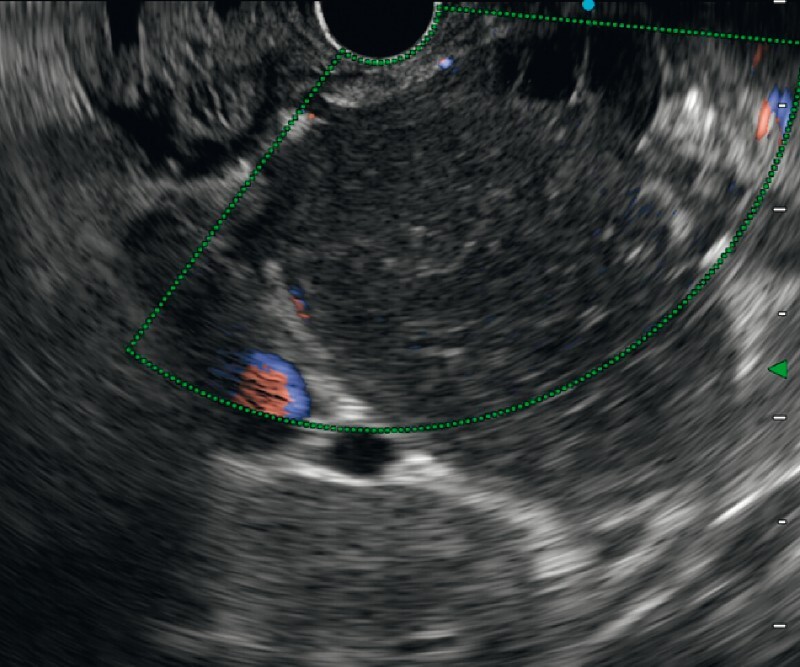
Endoscopic ultrasound appearance of cystic lymphangioma with vascularized walls.
